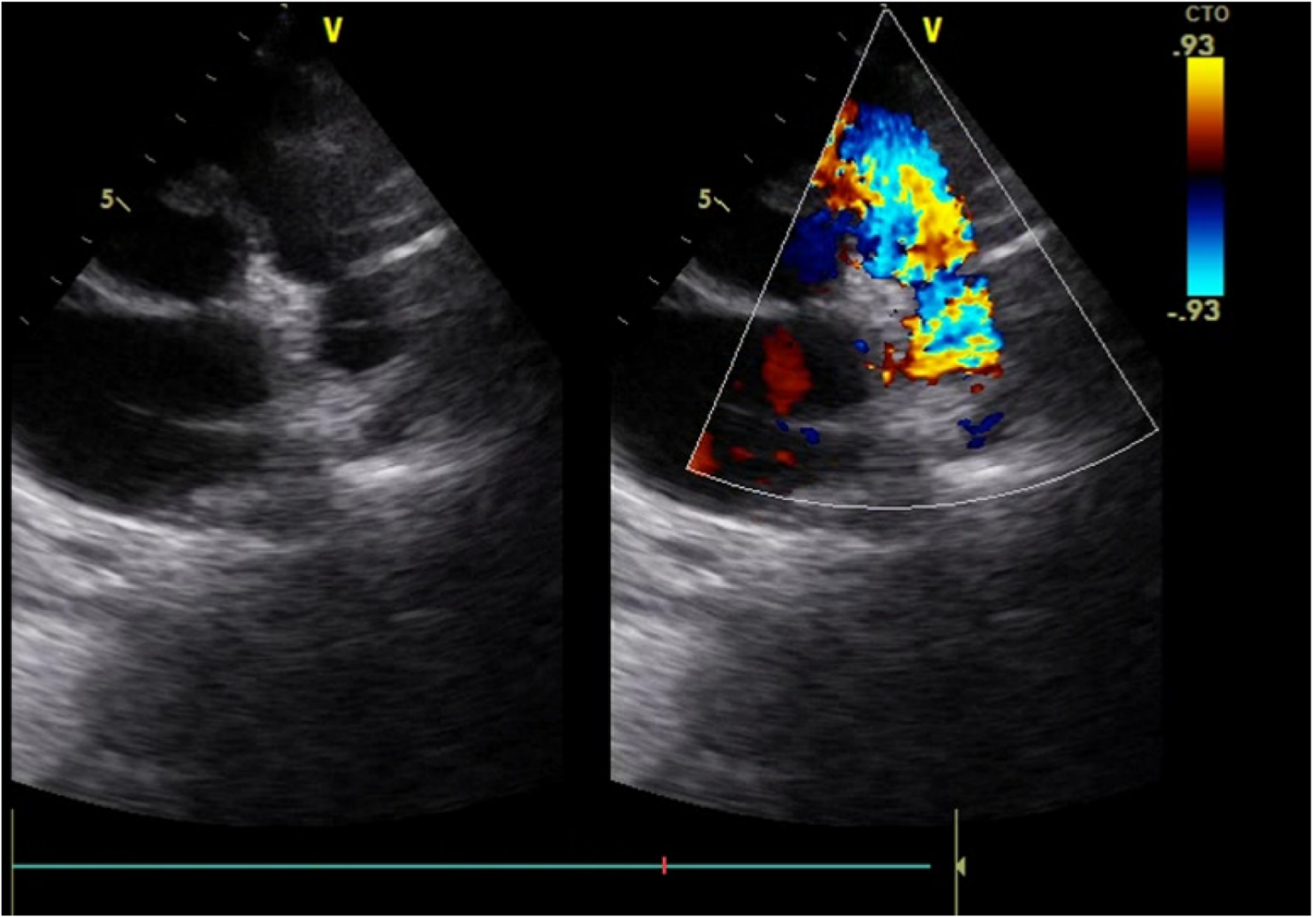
Parasternal short-axis view of a transthoracic echocardiogram with color Doppler showing an aliasing through the subpulmonic membrane
